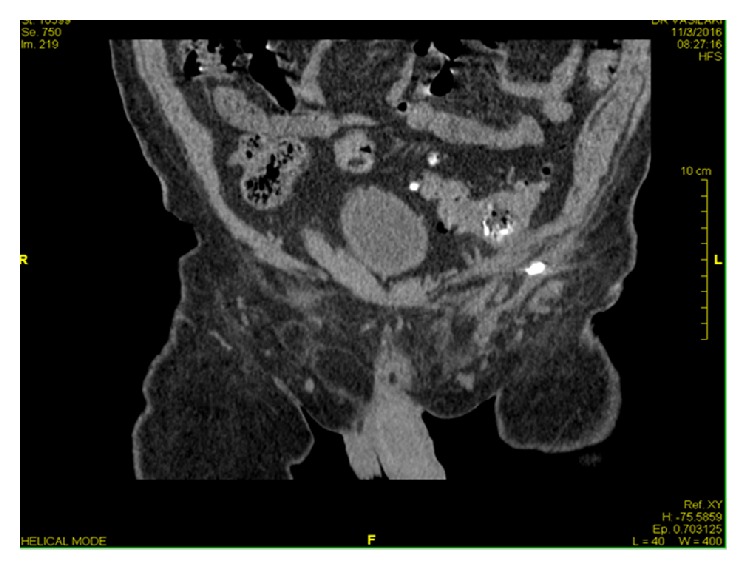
CT scan fistulogram confirming the colocutaneous fistula by the presence of contrast in the colon.
